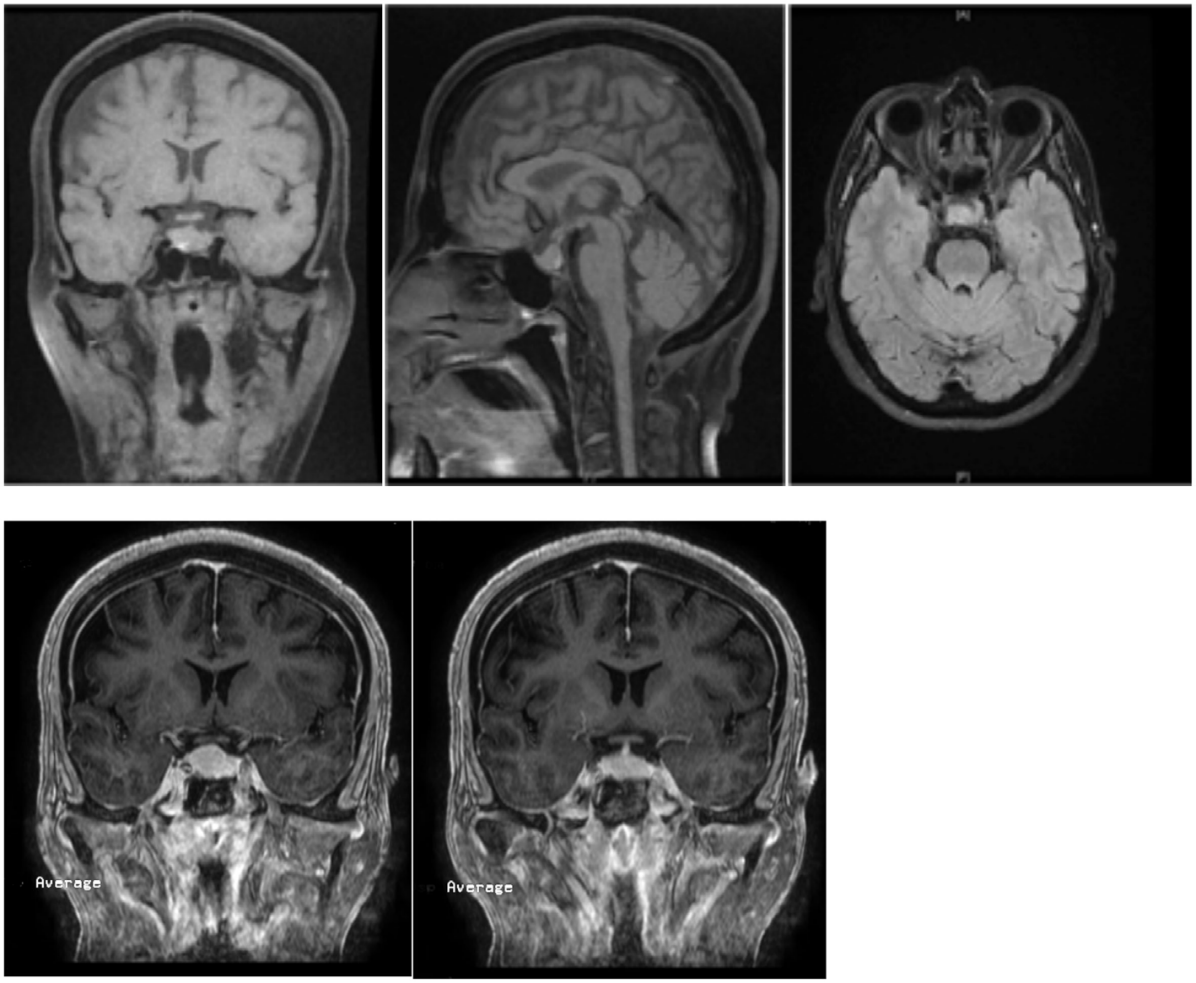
Repeated MRI once again showed a pituitary mass, measuring about 11 mm in height, which was reduced in size in comparison to the previous height of 18 mm. There was no compression of the optic chiasm or optic nerves. The pituitary stalk is in the center and no shift was noted.
